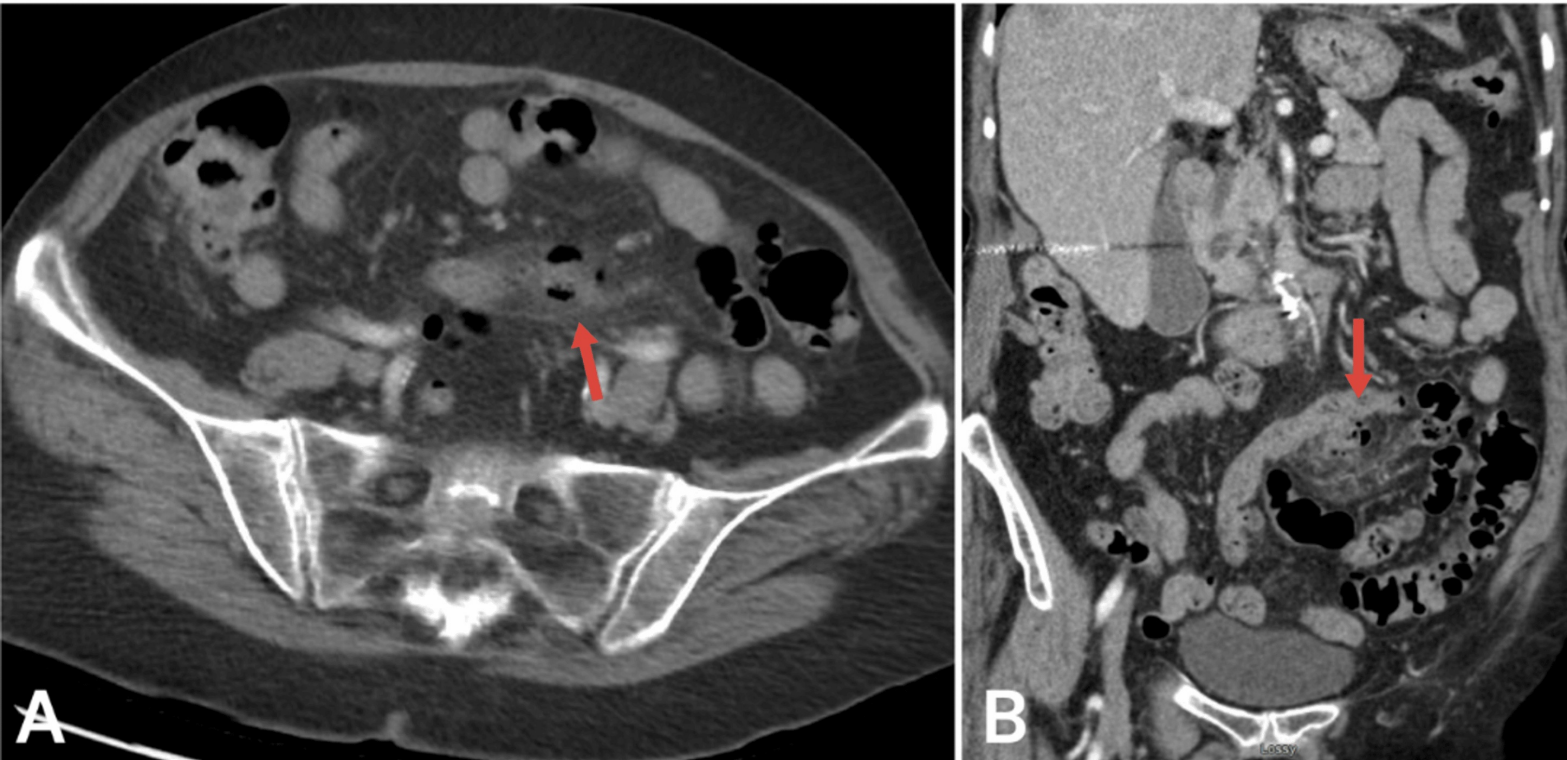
Abdomen and pelvis CT with intravenous IV contrast (a) Axial view of small bowel perforation and mesenteric air, (b) Coronal view of a small bowel diverticular perforation with significant fat stranding
